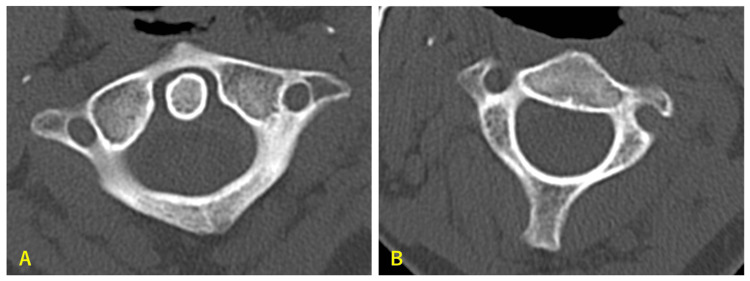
Axial scan using computed tomography (CT) The atlas is rotated approximately 20 degrees to the right (A) relative to the axis (B) and fixed. No expansion of the atlantodental interval (ADI) is seen.
